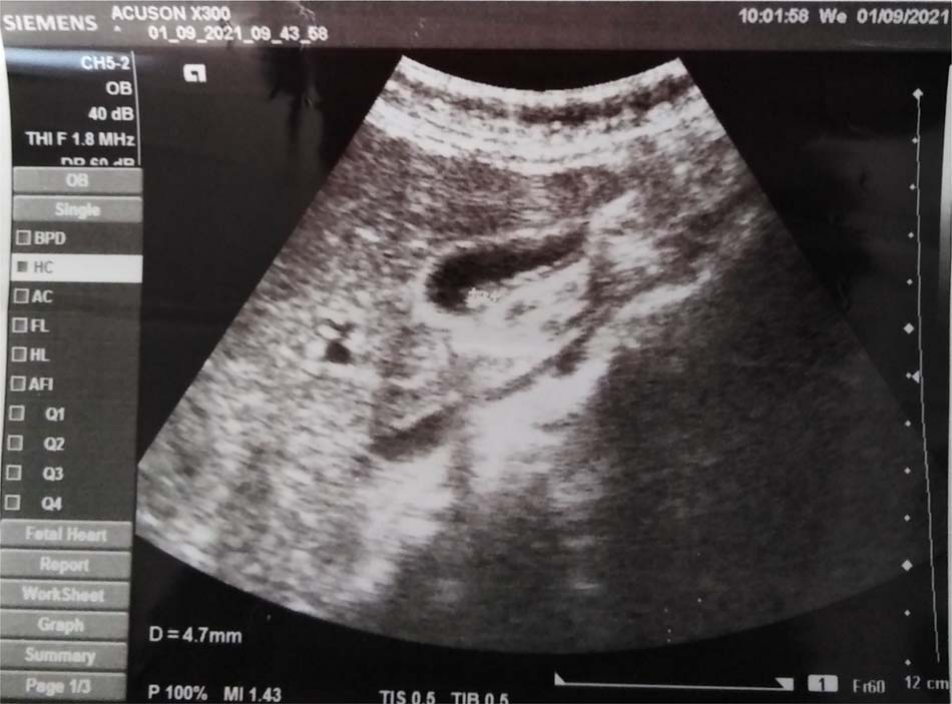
An ultrasonographic image of the gallbladder showed wall thickening with multiple gallstones in the lumen.
